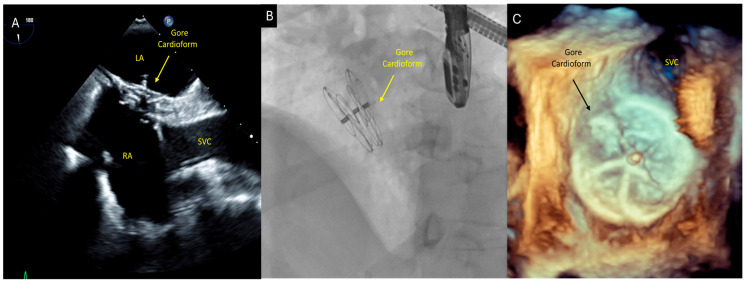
Percutaneous closure of the atrial septal defect. Given the presence of an ostium secundum ASD with right-sided chamber enlargement and the occurrence of a presumed embolic stroke without an alternative identified source, percutaneous closure was recommended following multidisciplinary discussion. The patient subsequently underwent successful percutaneous closure of the atrial septal defect using a 30 mm Gore Cardioform septal occluder device, achieving complete shunt resolution. TEE in the bicaval view (A) demonstrates the 30 mm Gore Cardioform septal occluder (W. L. Gore & Associates, Inc., Flagstaff, AZ, USA) well seated across the interatrial septum with stable positioning. A fluoroscopic image obtained immediately post-deployment (B) confirms correct device expansion and positioning. A three-dimensional en-face transesophageal echocardiographic view (C) provides detailed visualization of the occluder device in situ, confirming appropriate coverage of the defect and device stability. The patient recovered well with no recurrent neurological symptoms following ASD closure. Follow-up echocardiography demonstrated stable device position with no residual interatrial shunt. This case suggests presumed paradoxical embolism through an unrecognized atrial septal defect as a possible mechanism of postoperative stroke, after the exclusion of other identifiable causes. The diagnostic sequence—beginning with targeted neuroimaging, followed by contrast-enhanced transthoracic echocardiography and definitive transesophageal assessment—highlights the essential role of multimodality imaging in identifying occult interatrial communications in adult patients presenting with embolic stroke of undetermined origin. LA = left atrium; RA = right atrium; SVC = superior vena cava.
